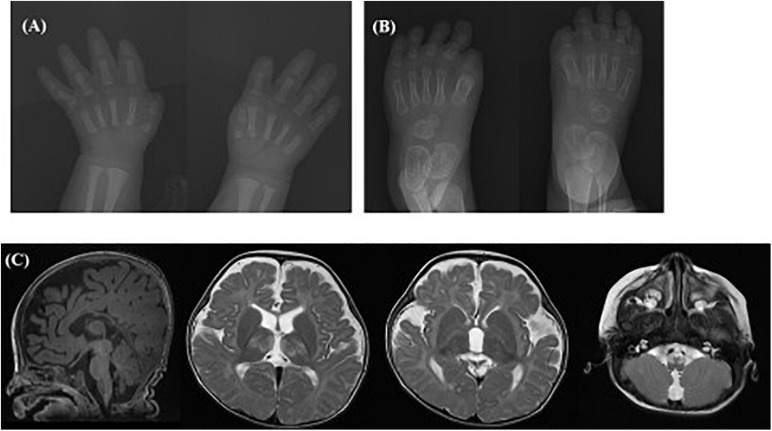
Radiographic images of case #30 with Yunis-Varon dysplasia. X-ray showing hypoplasia and agenesis of the distal phalanx of the (A) fingers and (B) toes. (C) Brain MRI showing diffuse corpus callosal thinning, prominent subarachnoidal space at the frontotemporal convexity, and dilation of the 3rd ventricle, T2 high signal intensity of the bilateral thalami and medulla oblongata of inferior olivary nuclei.
